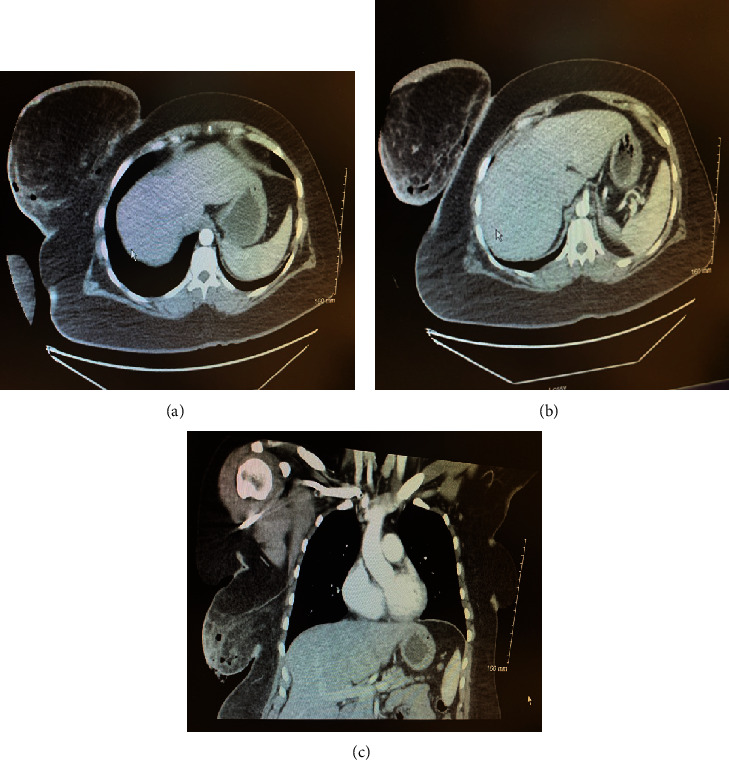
(a, b) Axial view of Chest CT depicting increased inflammation of the right breast and associated locules of gas along lateral and posterior aspect of breast. (c) Coronal view of Chest CT image showing increased attenuation of the right inferolateral breast tissue with several locules of gas. Images obtained with patient permission.
